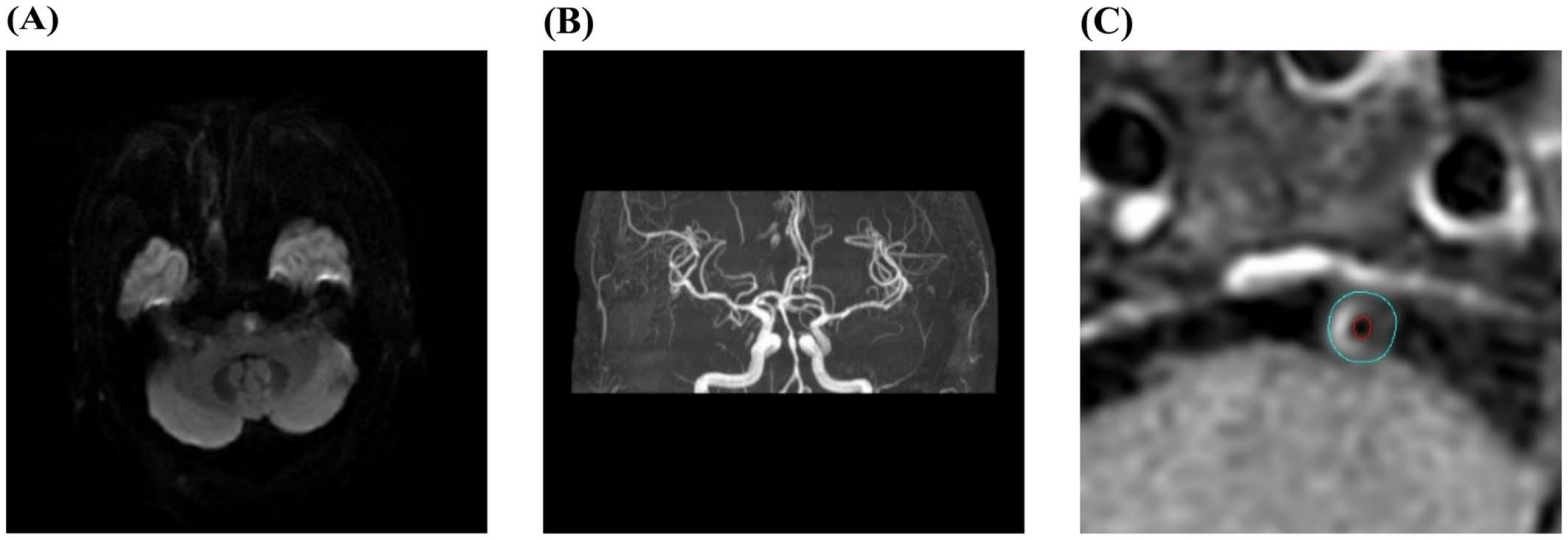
Typical images of contrast-enhanced high-resolution vessel wall imaging in a sICAS patient. (A–C) Male, 56 years old, with right-sided limb weakness for 1 day. DWI showed acute infarction in the left pons (A); MRA showed severe stenosis of the basilar artery (B); HR-VWI enhanced image showed an enhanced plaque in the basilar artery. The blue line outlined the vessel wall area, and the red line outlined the lumen area (C).
